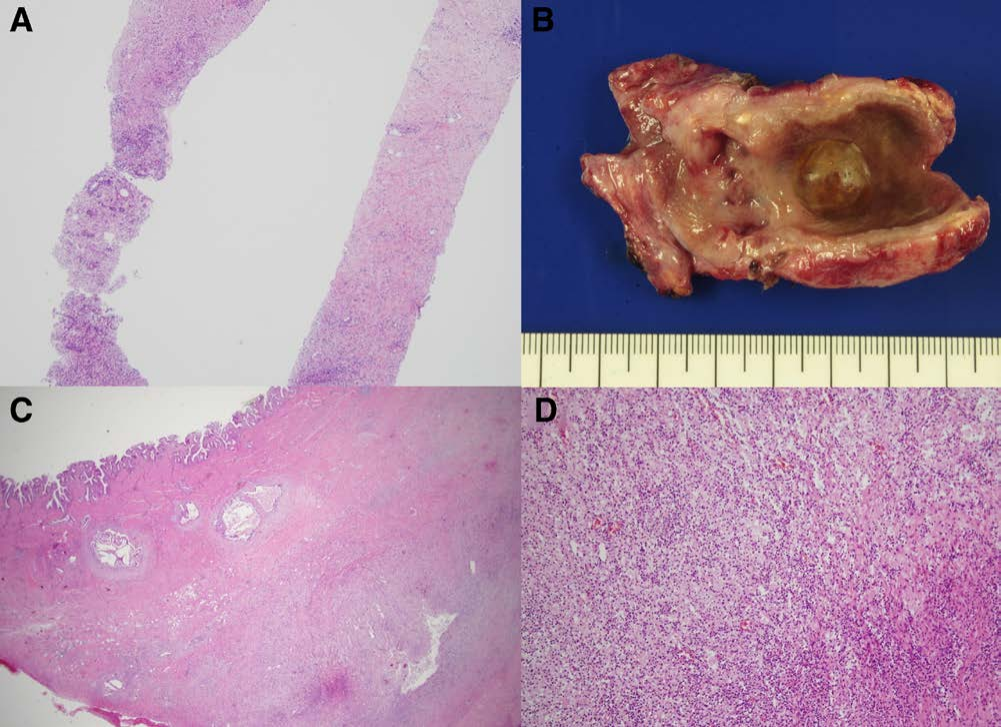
Axial and coronal computed tomography (CT) images of a gallbladder lesion. Marked gallbladder wall thickening with intramural low-density areas was noted along with obscured fat planes between the gallbladder and transverse colon, raising suspicion of complicated cholecystitis or malignancy. These findings prompted an image-guided percutaneous biopsy.
